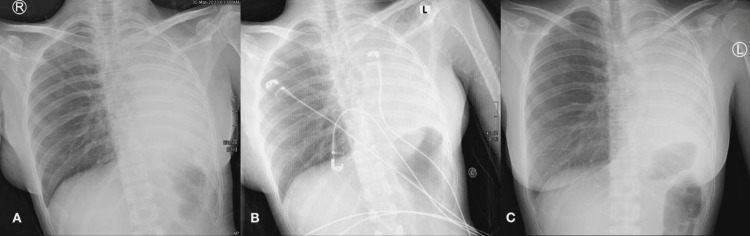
Chest X-ray showing complete left lung collapse.
